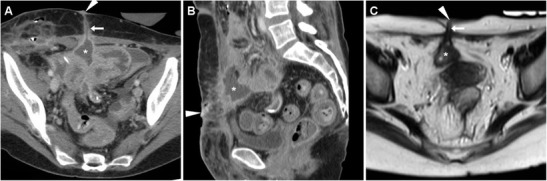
Two examples of postsurgical fistulas draining intra-abdominal collections, without bowel involvement. In a 34-year-old woman, after ileocecal resection CT (A, B) showed an EFO (arrowheads) communicating via a “tram-track” fistula (arrows) through the abdominal wall with a peripherally enhancing collection (*) which ultimately resolved. In a 48-year-old woman with recent CD resection, T2-weighted MRI (C) depicted a chronic postsurgical collection (*) connected to the skin by a fistula (arrows)
